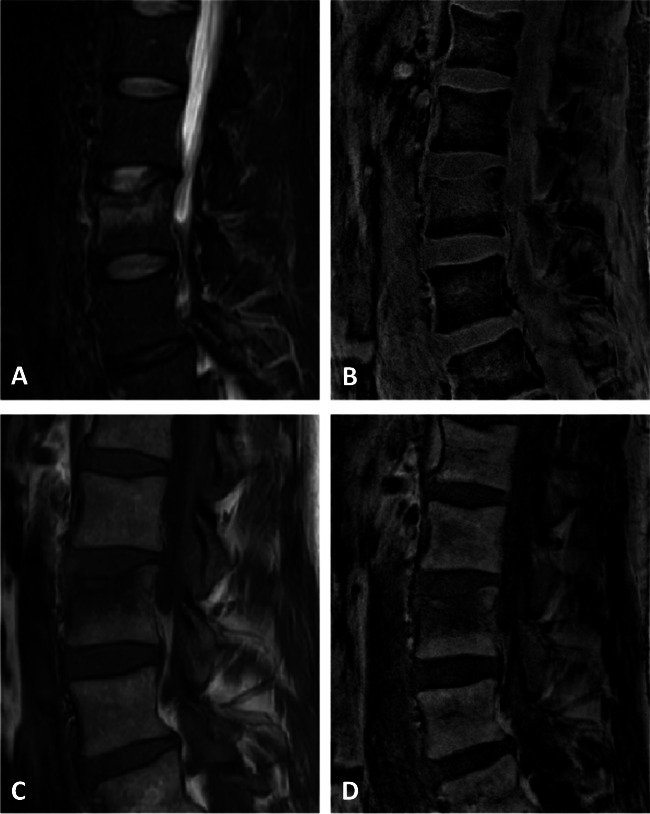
Standard short-tau inversion recovery sequence image (STIR) showing a bone marrow edema of an acute fracture of the second lumbar vertebra (A). UTE-derived water-separated image showing an edema equivalent to the STIR sequence (B). Standard T1-weighted sequence image (C) and correlating to UTE-derived fat-separated image (D) both showing a signal reduction within the fractured vertebra
